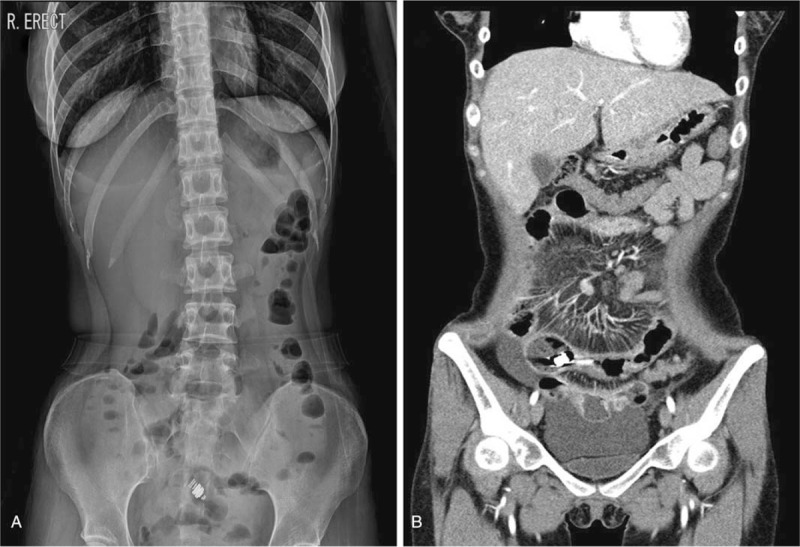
Abdominal radiograph and computed tomography scan 11 months after capsule endoscopy. (A) Stepladder sign and capsule retention are identified in the lower abdomen. (B) Multifocal wall thickenings and luminal narrowing are observed in the ileum, and capsule retention is identified near the luminal narrowing.
